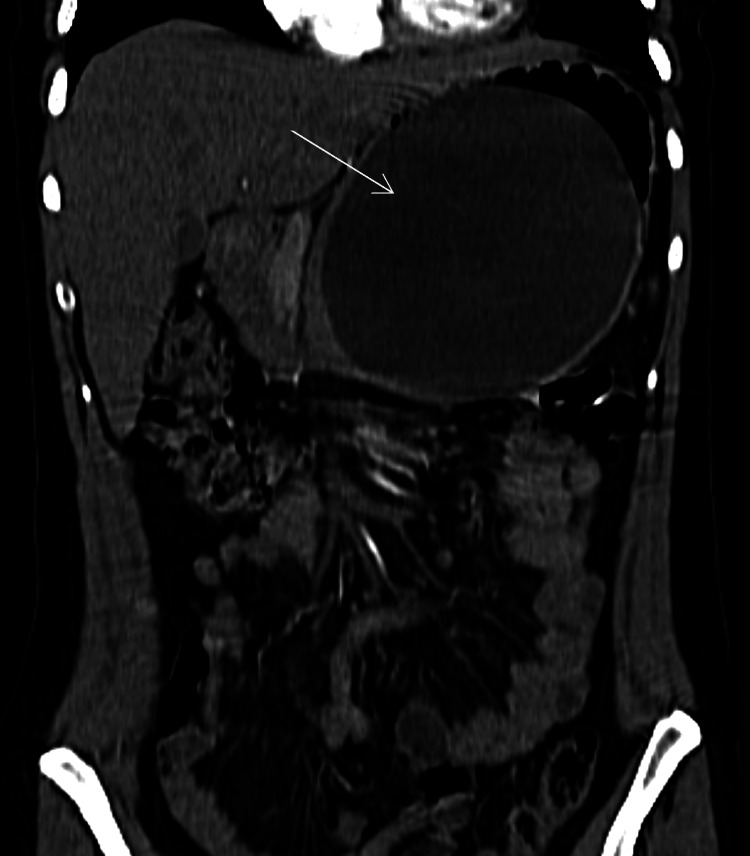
Computed tomography scan of the abdomen in the coronal plane, showing the over-distended intragastric balloon in its correct gastric location (white arrow).
